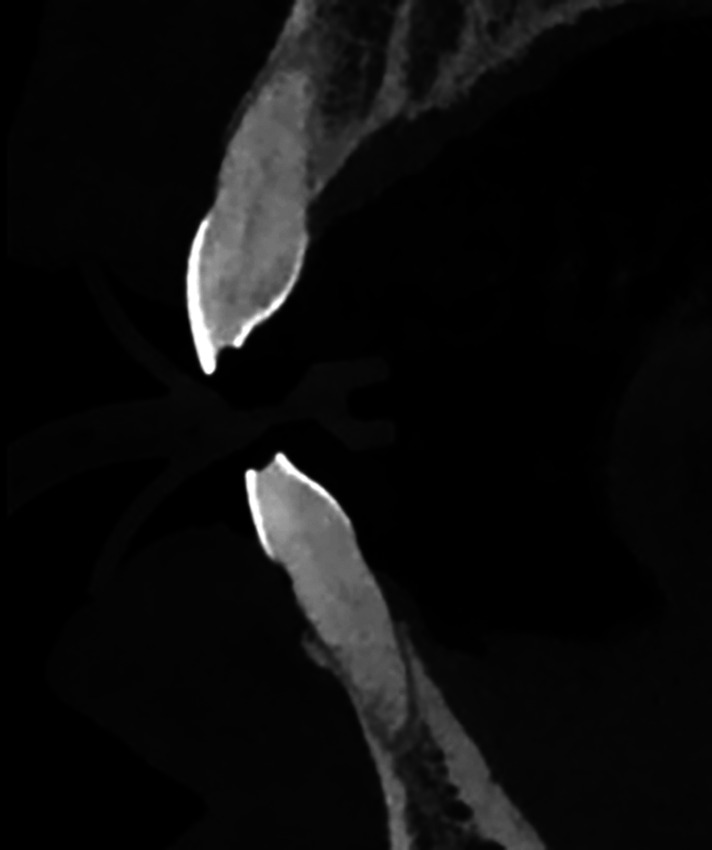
Occlusal wear visualization on CBCT.
